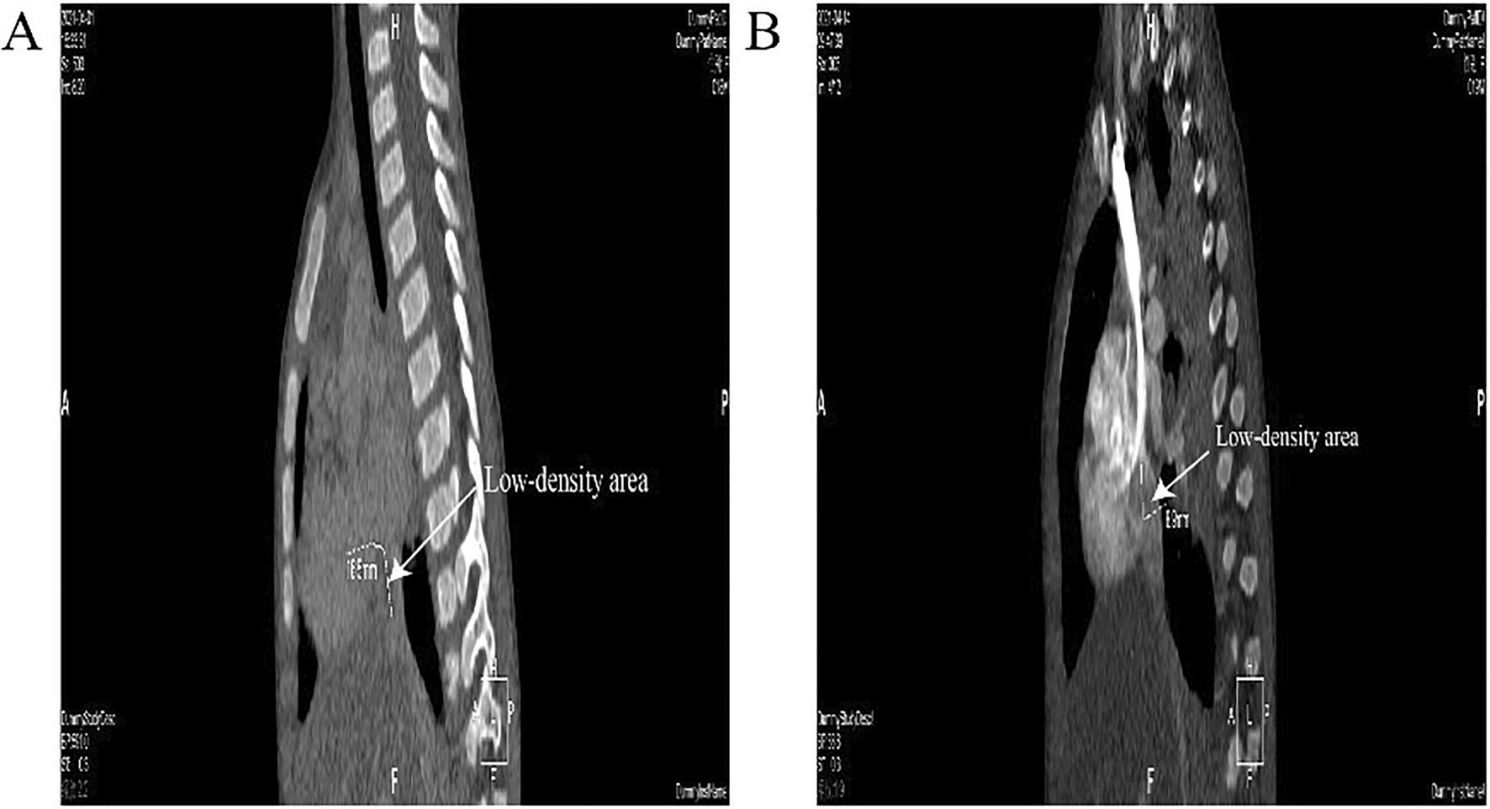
CTA images during the patient's course of disease. (A) The CTA image of April 1 shows the presence of low‐density area. (B) The CTA image of April 14 shows the presence of low‐density area in the right atrium. CTA, computed tomography angiography.
